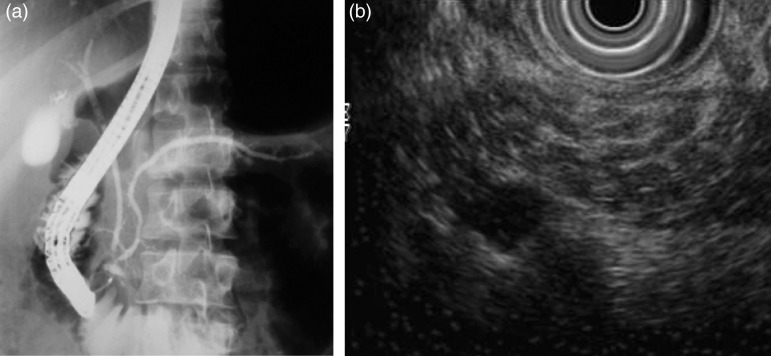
(*a*) Subtle changes of early chronic pancreatitis in the main pancreatic duct on endoscopic retrograde cholangiopancreatography (ERCP). (*b*) Pancreatic parenchymal changes in the form of honeycombing and hyperechoic foci suggestive of early chronic pancreatitis on endoscopic ultrasonography (EUS).
